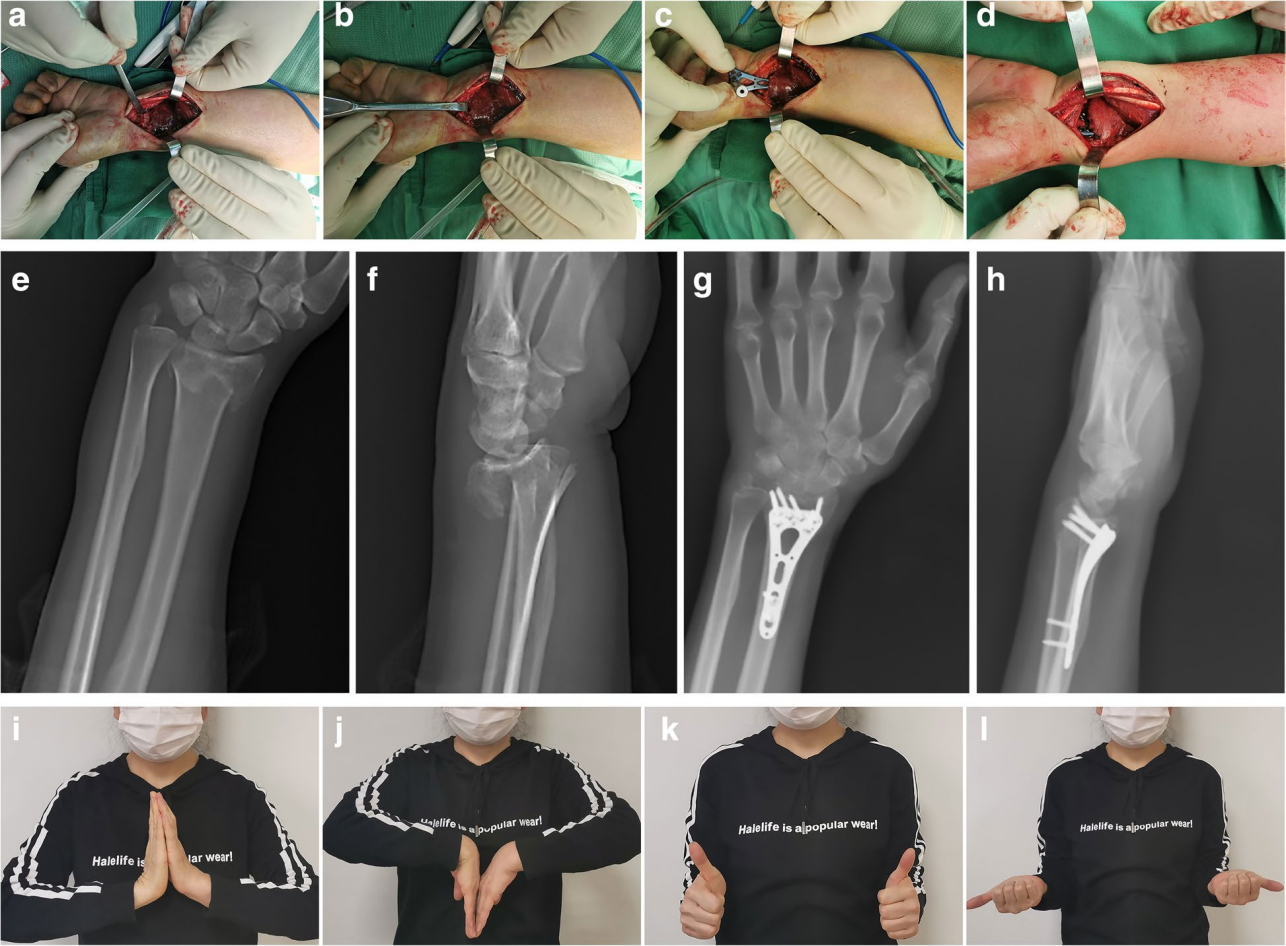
a 55-year-old woman with a fall injury resulting in a left distal radius fracture of type B2 according to AO classification. b A tunnel was established under the PQ muscle during the operation. c The plate was placed below the PQ muscle. d Pre-incisional closure macrophotograph. e–f Preoperative x-ray of the affected limb. g-h Postoperative x-ray of the affected limb. i-l Wrist function at 3 months after surgery
